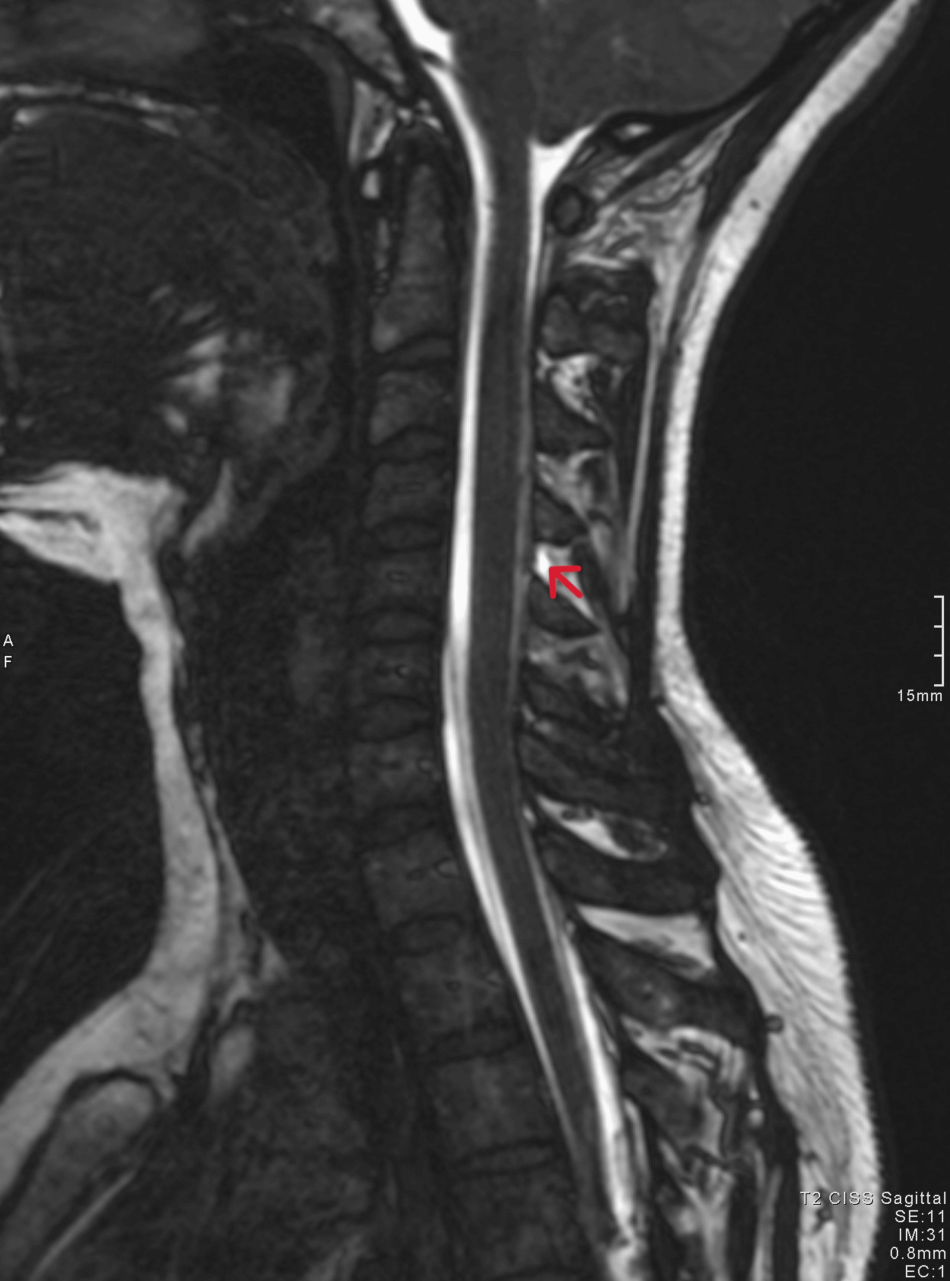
MRI constructive interference in steady-state sequence sagittal view showing fluid signal at the level of the C4/C5 interspinous process.
